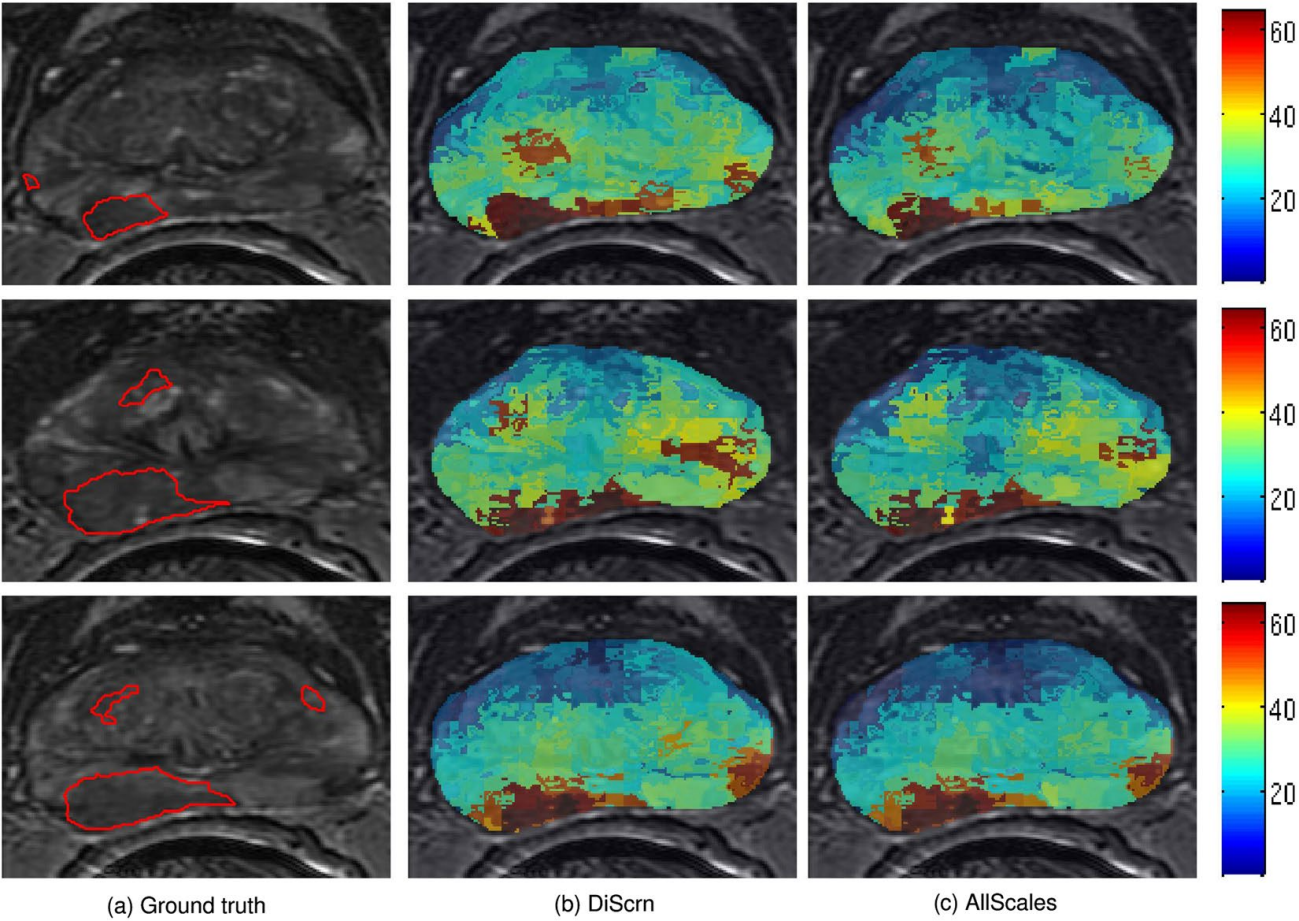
The learned scale weights (mean + std) of (a) Gabor, (b) Haralick, (c) LBP, and (d) PHOW on MRI images from the experiments of E1 (D1), E2 (D2), E3 (D1 + D2), E4 (D1 → D3) and E5 (D3 → D1). The horizontal axis represents the used three feature scales (i.e., 3 × 3, 5 × 5, 7 × 7) for the five different experiments. The vertical axis represents the learned weights. High weight indicates high importance and vice versa.
